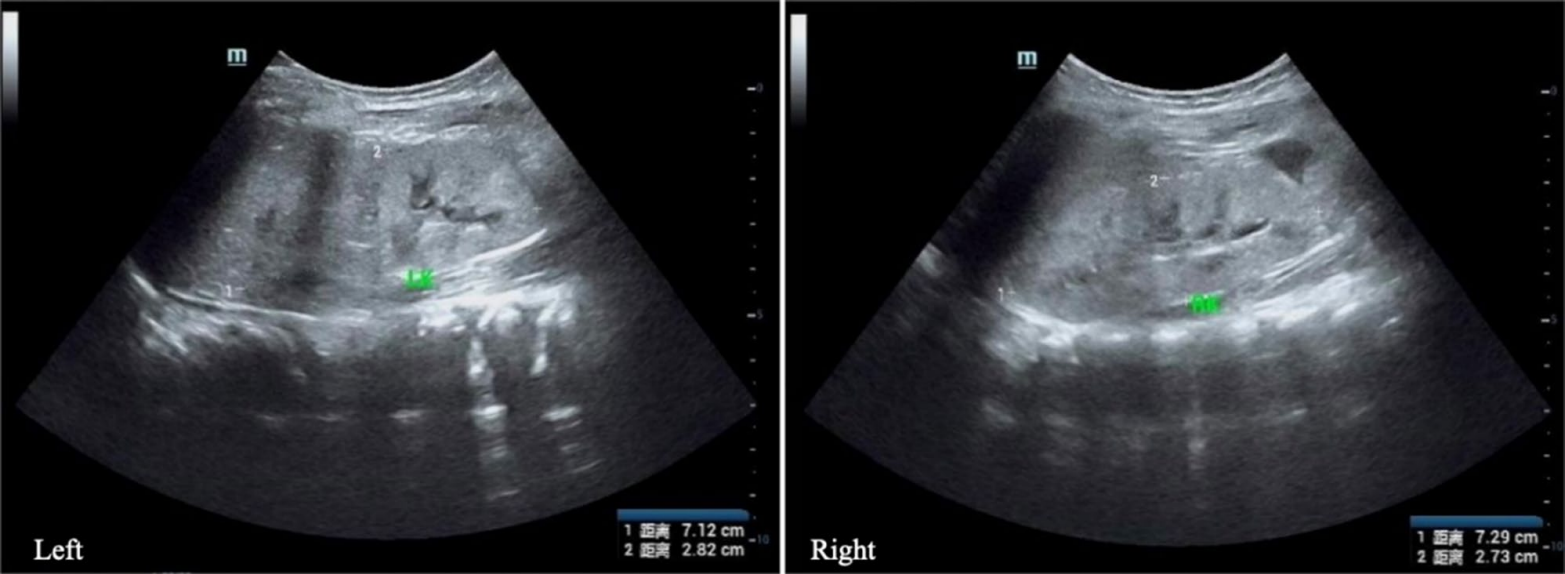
Ultrasound examination of the kidneys. Ultrasound examination shows that the size of the left kidney is about 7.1 × 2.8 cm, and the size of the right kidney is about 7.3 × 2.7 cm. The parenchymal echo of both kidneys is enhanced, and the boundary between the upper cortex and medulla of the right kidney is unclear
